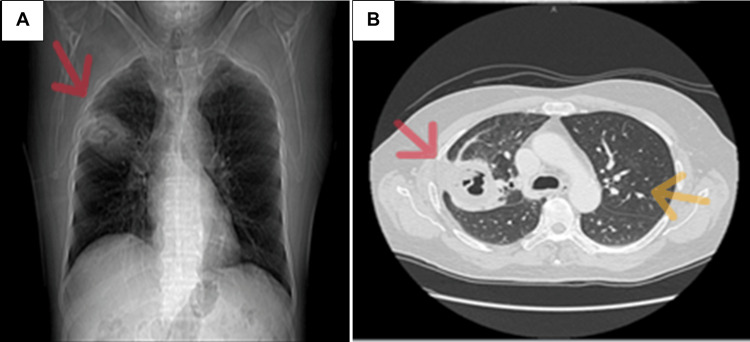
Initial chest radiograph and CT scan showing cavitary lesion and pulmonary nodules. (A) Chest X-ray demonstrating a right upper lobe cavitary opacity with associated volume loss. (B) Contrast-enhanced computed tomography (CT) of the thorax confirming a cavitary lesion in the right upper lobe measuring 5.3 × 4.8 cm with adjacent pleural reaction (red arrows). Also noted are a 6 mm subpleural nodule in the left lateral lung and a 4 mm nodule in the left upper lobe (yellow arrows).
